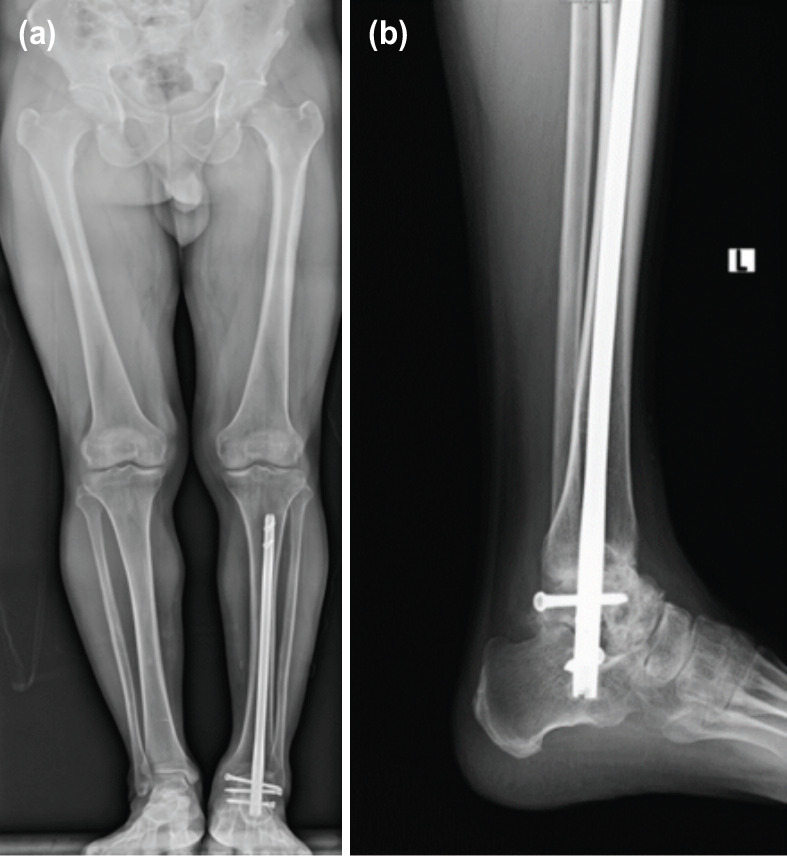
(a, b) AP and lateral radiographs of Patient 4 at follow-up of 12 months.
